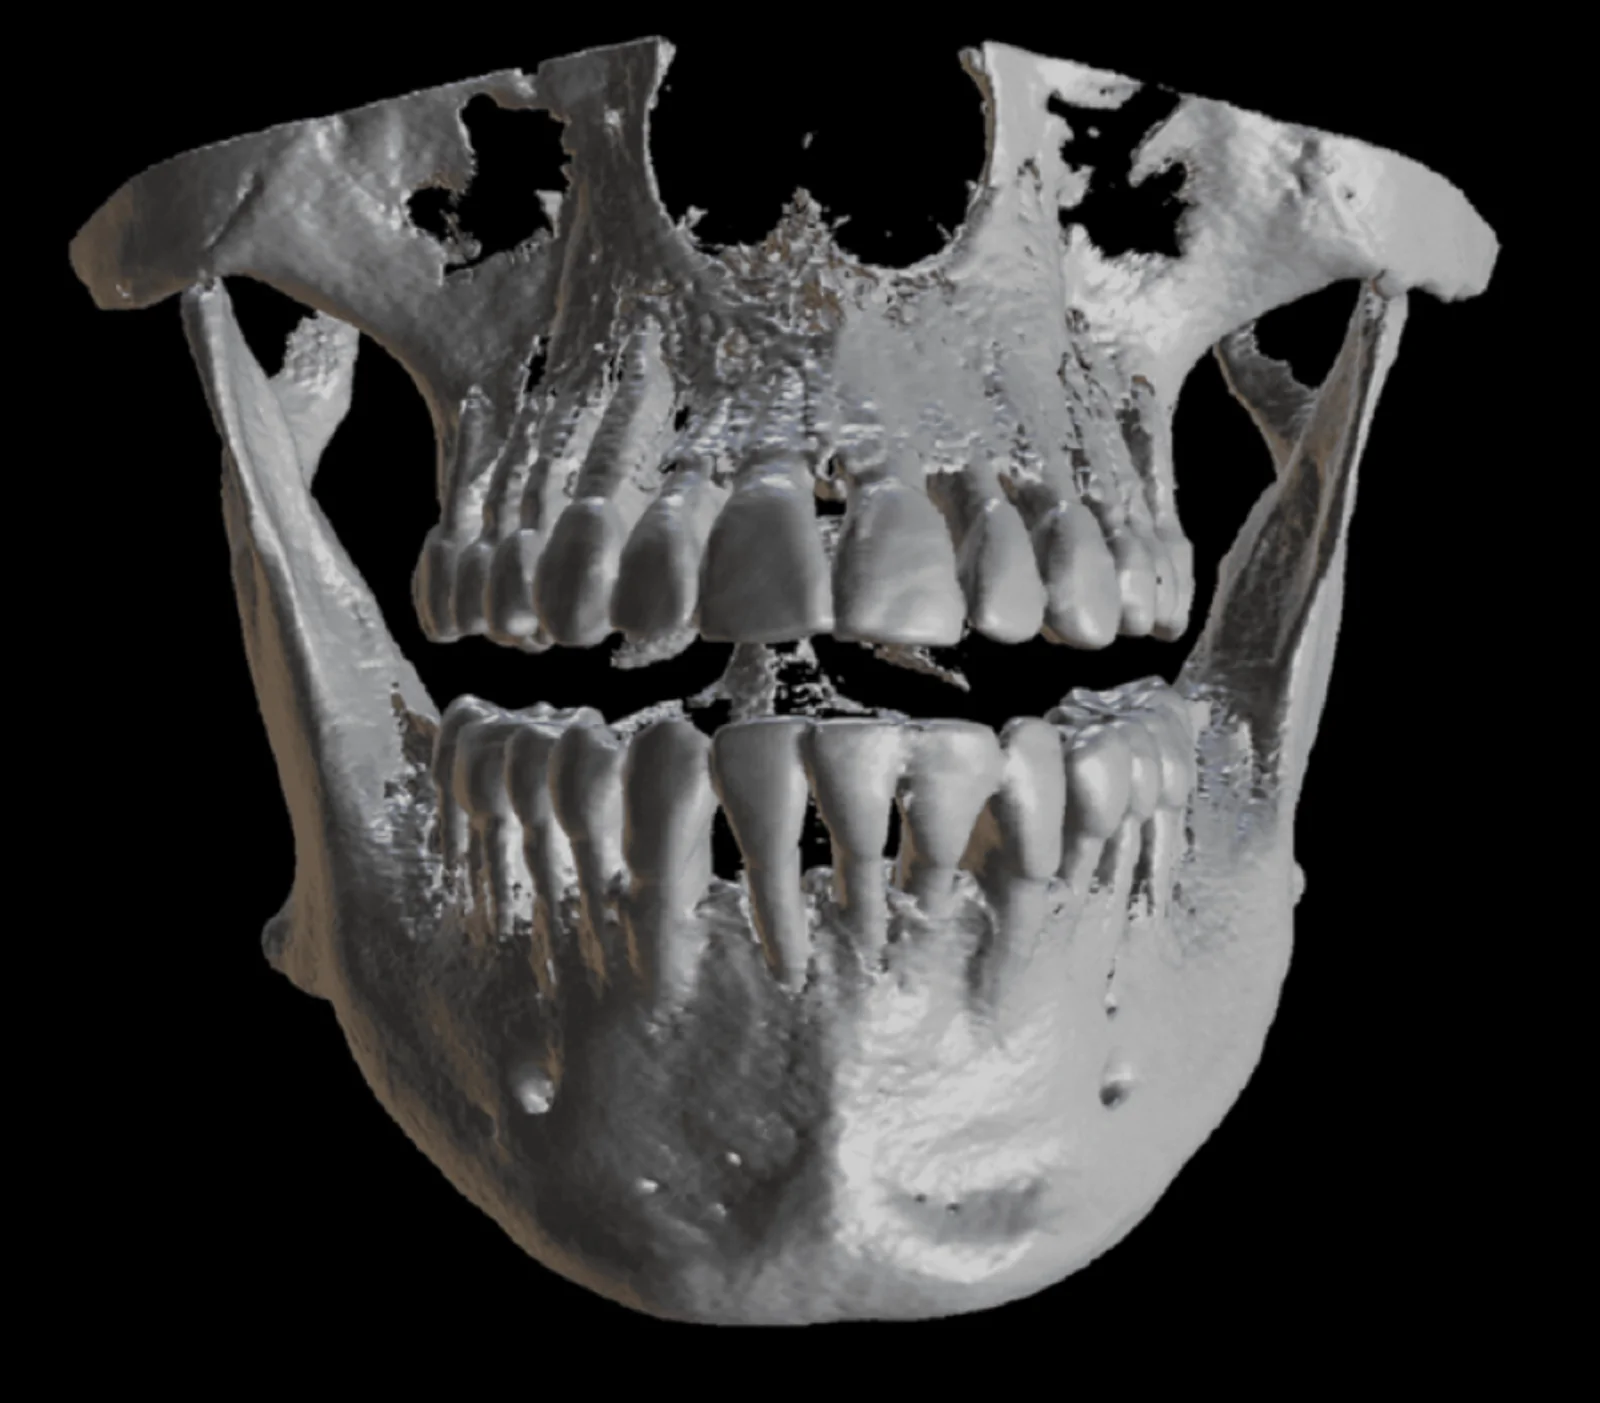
Three-dimensional CBCT reconstruction of the mandibular anterior region. Three-dimensional cone-beam computed tomography (CBCT) reconstruction demonstrating reduced facial alveolar bone support in the mandibular anterior region, with areas of facial alveolar bone loss (dehiscence) associated with the teeth affected by gingival recession. These findings supported the diagnosis and assisted in planning root coverage using a connective tissue graft and a coronally advanced flap.
